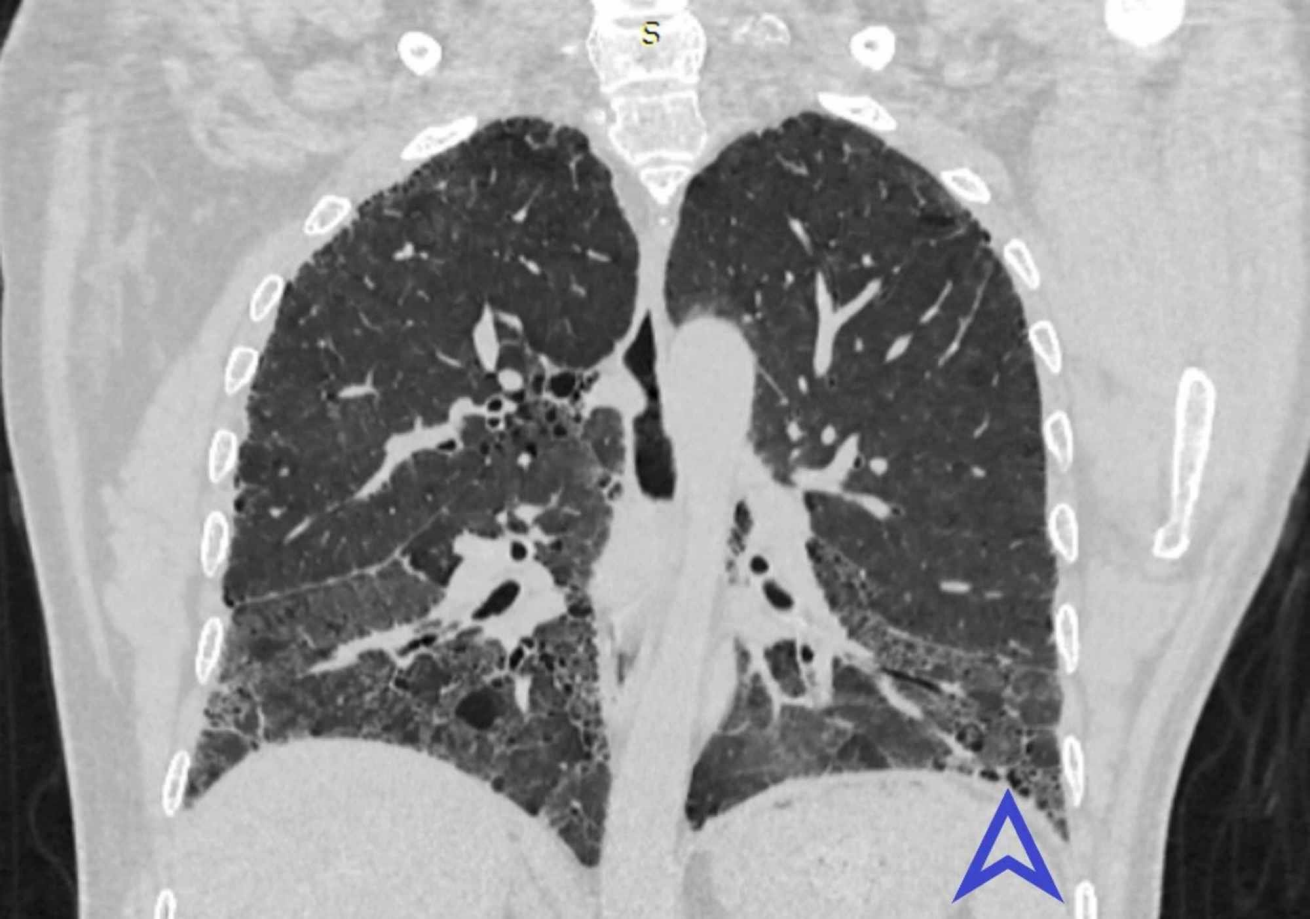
Coronal view HRCT shows bilateral symmetrical fibrosis in lower lobes HRCT - high-resolution computed tomography Blue arrowhead shows honeycombing.
